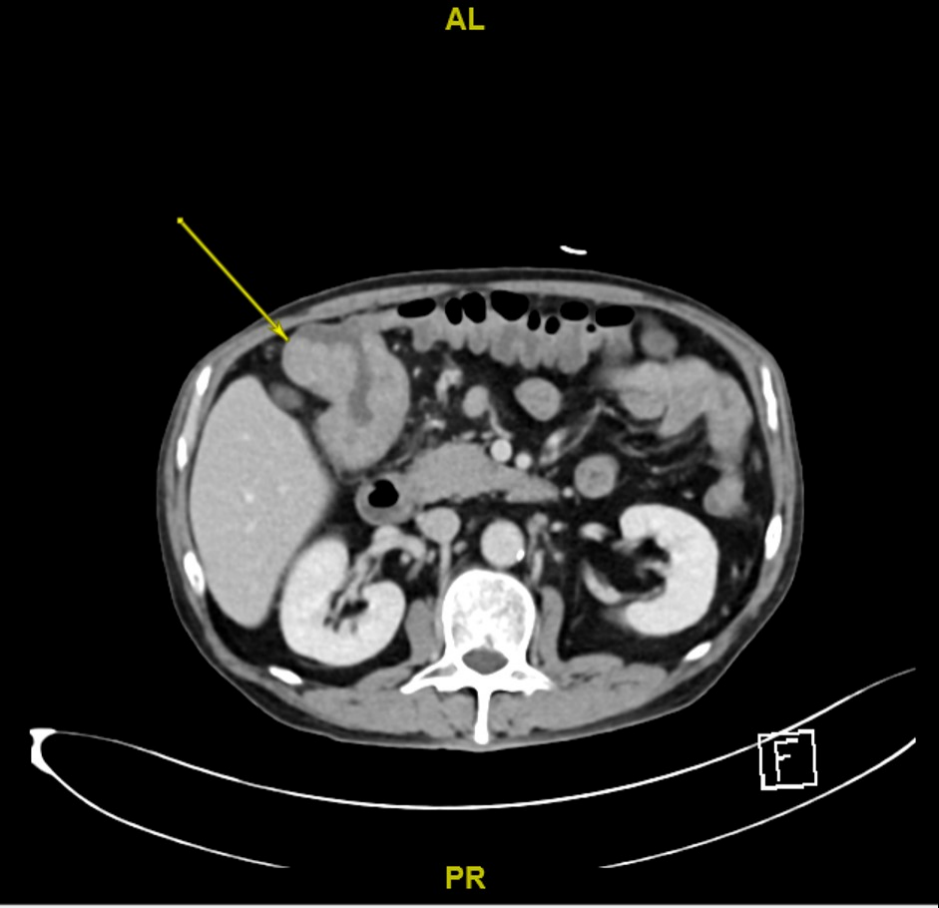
Axial CT scan showing a thickened loop of colon in the right hypochondrium.
